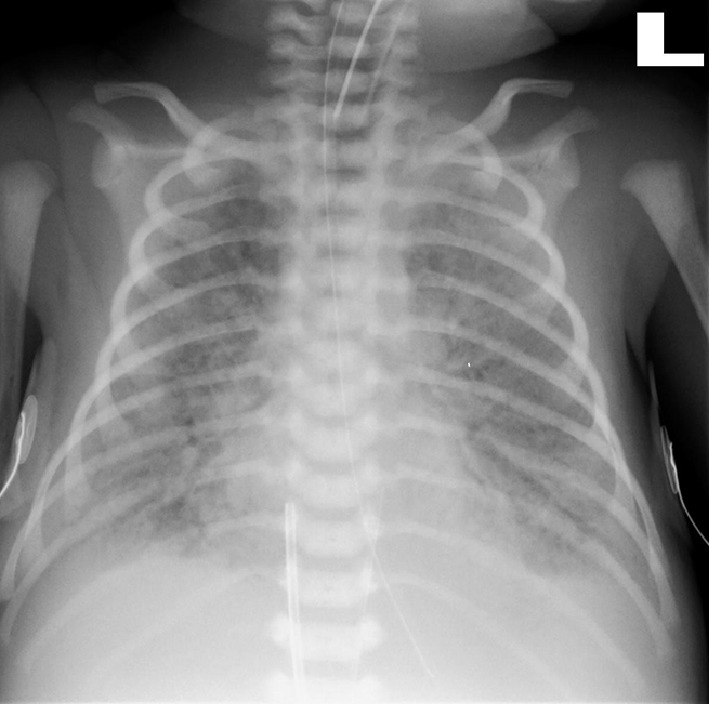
Chest X-ray on day 4. Diffuse alveolar pattern.
